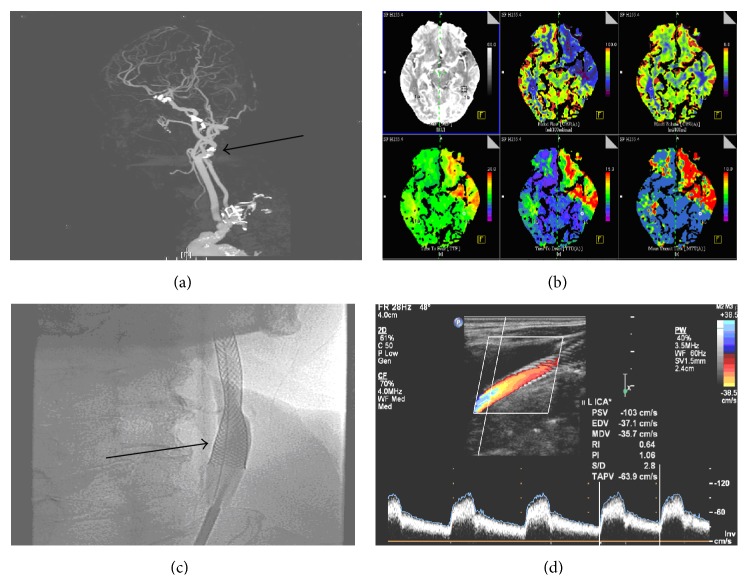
An example of left side severe internal carotid artery stenosis undergoing carotid stenting treatment. An 85-year-old male patient presented with right side hemiparesis and aphasia 24 hours before admission to our neurology ward. (i) (a) denotes CTA scan showing >70% lumen reduction of left side proximal internal carotid artery and distal part of common carotid artery (black arrow). (ii) (b) shows the CT perfusion scan that suggests decreased blood perfusion to the left cerebral hemisphere indicating critical blood flow demand compared to the right side cerebral hemisphere. (iii) (c) presents the poststenting status with umbrella device that prevent embolus distal migration causing major subsequent stroke event (black arrow). (iv) (d) displays the follow-up cervical carotid ultrasound of normalized flow profile.
